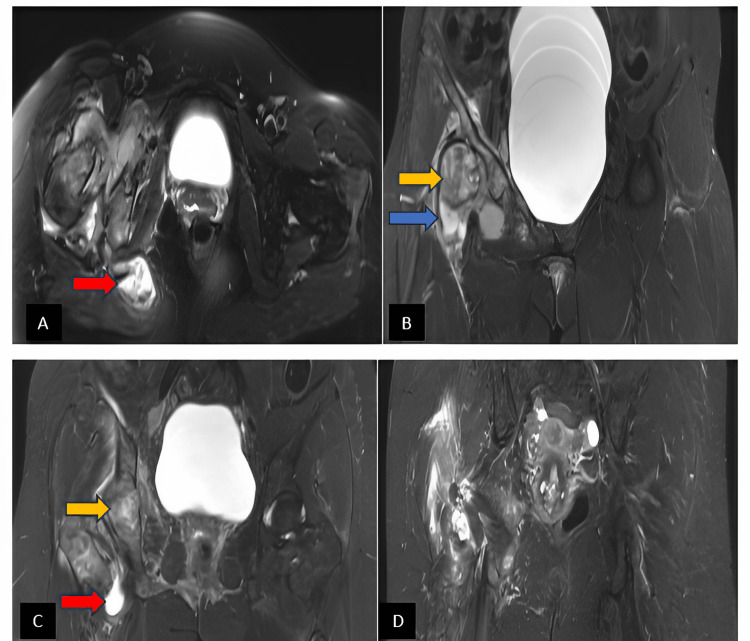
MRI of the right hip with contrast: axial view in T2WI (A), coronal view in T2WI (B), T2WI with contrast (C), and T2W1 of the right hip (D). There is effusion with synovial thickening of the right hip showing enhancement after contrast (blue arrow) and diffuse bone marrow edema involving the right acetabulum (yellow arrow). A large focus anterior to the right gluteus maximus muscle medially measuring 3.5 × 2.7 cm shows increased signal intensity on T2 with another focus adjacent to the right acetabular labrum measuring 2.8 × 1.7 cm slightly hyperintense on T2, representing collections (red arrow). MRI: magnetic resonance imaging, T2WI: T2-weighted image
